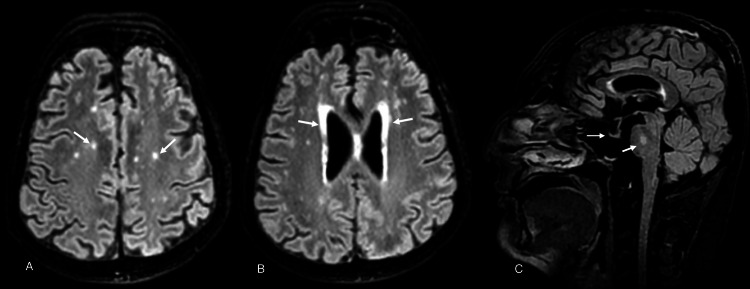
Brain MRI of Patient 2 (A) Multiple and bilateral subcortical FLAIR hyperintensities; (B) confluent bilateral periventricular white matter T2/FLAIR hyperintensities; (C) shallow sella turcica with a small pituitary gland (thin arrow) with central pontine FLAIR hyperintensity (thick arrow).
